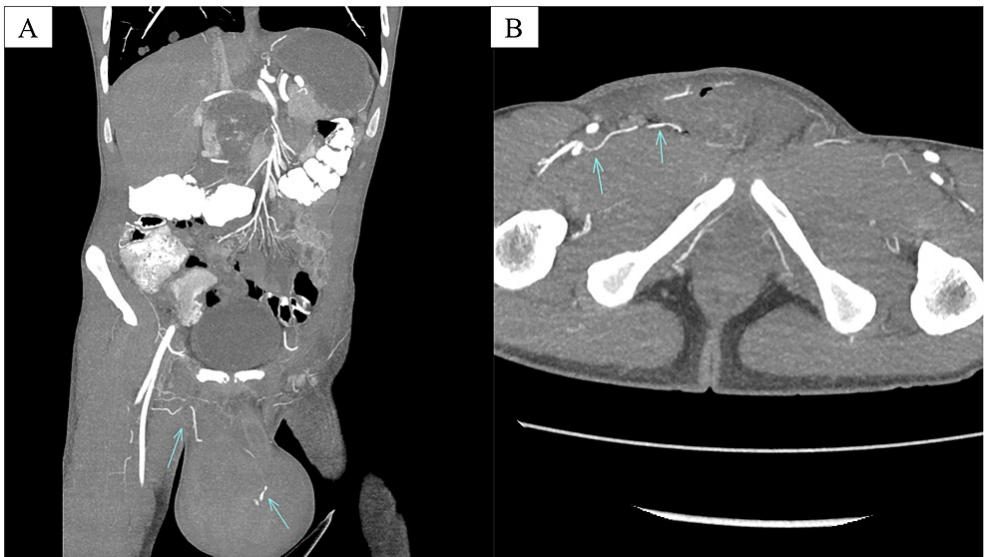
Coronal (A) and axial (B) maximal intensity projection images in the arterial phase from the contrast-enhanced CTA of the abdomen and pelvis demonstrating a large heterogenous collection within the right scrotum consistent with a hematoma, with evidence of active contrast extravasation. The arterial supply can be traced to the profunda femoris artery (arrows). CTA: computed tomography angiography
